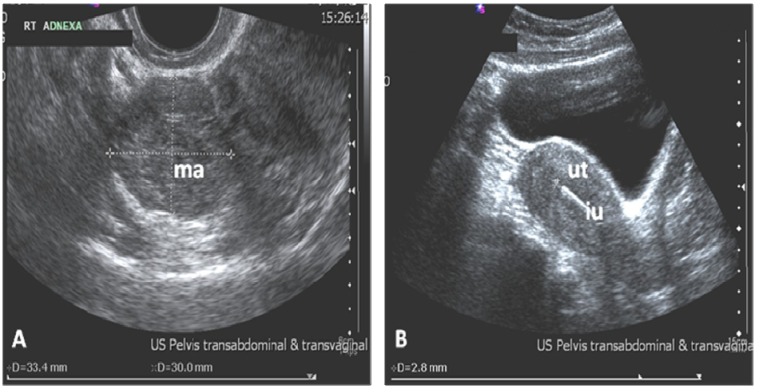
Ultrasound image demonstrating a mass (ma) in the right adnexa (A); (B) shows a thin endometrium with a normally sited intrauterine contraceptive device (IUD) (iu) in the uterus (ut).
